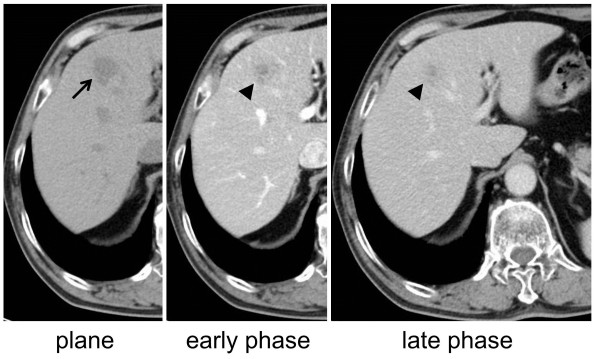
Computed tomography (CT) findings nine years after the first surgery. A 14-mm low-density area (arrow) in segment 8 of the liver (plane) is observed. The periphery of this area was nonhomogeneously enhanced by contrast medium and appeared to be isodense in the late phase (arrow head) compared to surrounding normal liver tissue.
